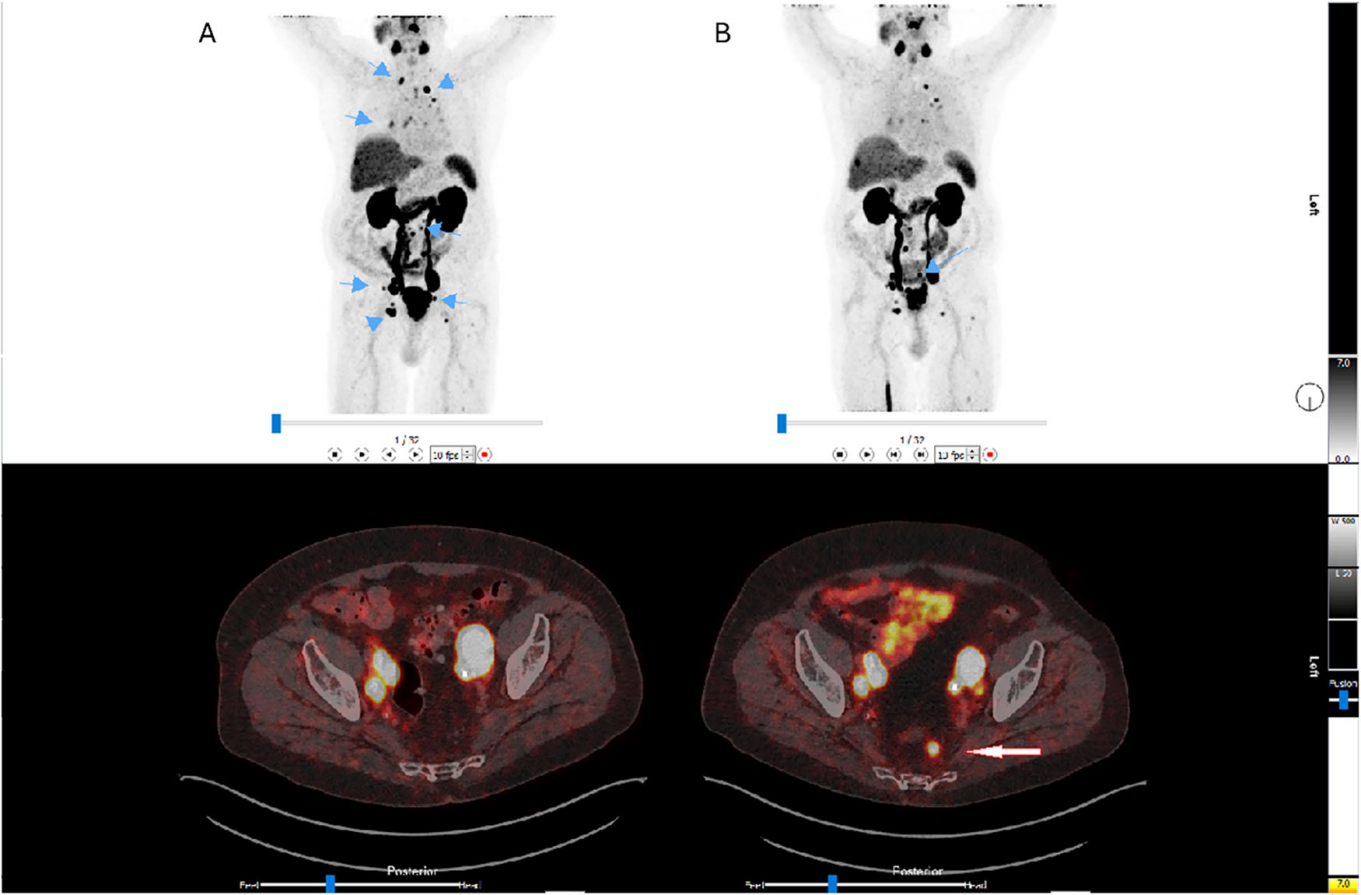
Early interim MIPs and [^68^Ga]Ga-PSMA-11 PET-CT performed 2 cycles post-treatment (blue arrow points to a reduced area of radiotracer uptake, suggesting partial metabolic response or residual disease at that site. Most previously PSMA-avid lesions have resolved or show significantly lower uptake (B), and pretreatment (blue arrows pointing to multiple PSMA-avid metastatic lesions) (A). New posterior pelvic lesion despite PSA drop from 16.36 ng/mL to 9.65 ng/mL. Courtesy of Drake B
